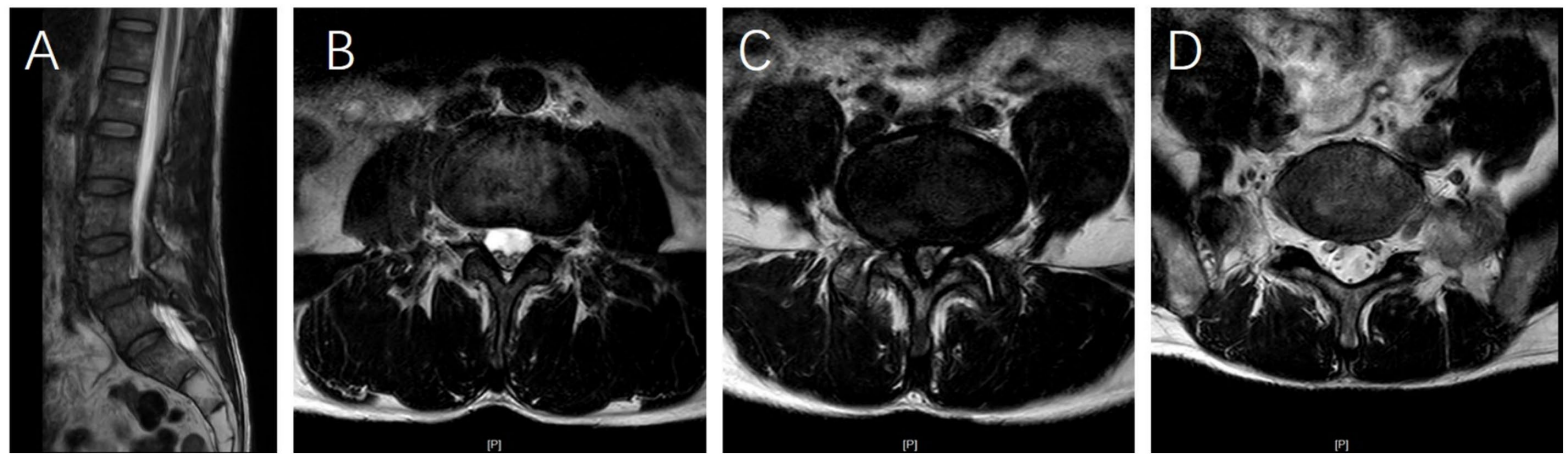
(A) Sagittal T2-weighted magnetic resonance imaging (MRI) of a 62-year-old female patient demonstrating L4 vertebral spondylolisthesis. (B–D) Axial T2-weighted MRI scans at the L3/4, L4/5, and L5/S1 levels reveal that multifidus muscle atrophy (MMA) is most severe at the L4/5 level compared to the L3/4 and L5/S1 levels, whereas the degree of MMA is similar between the L3/4 and L5/S1 levels.
